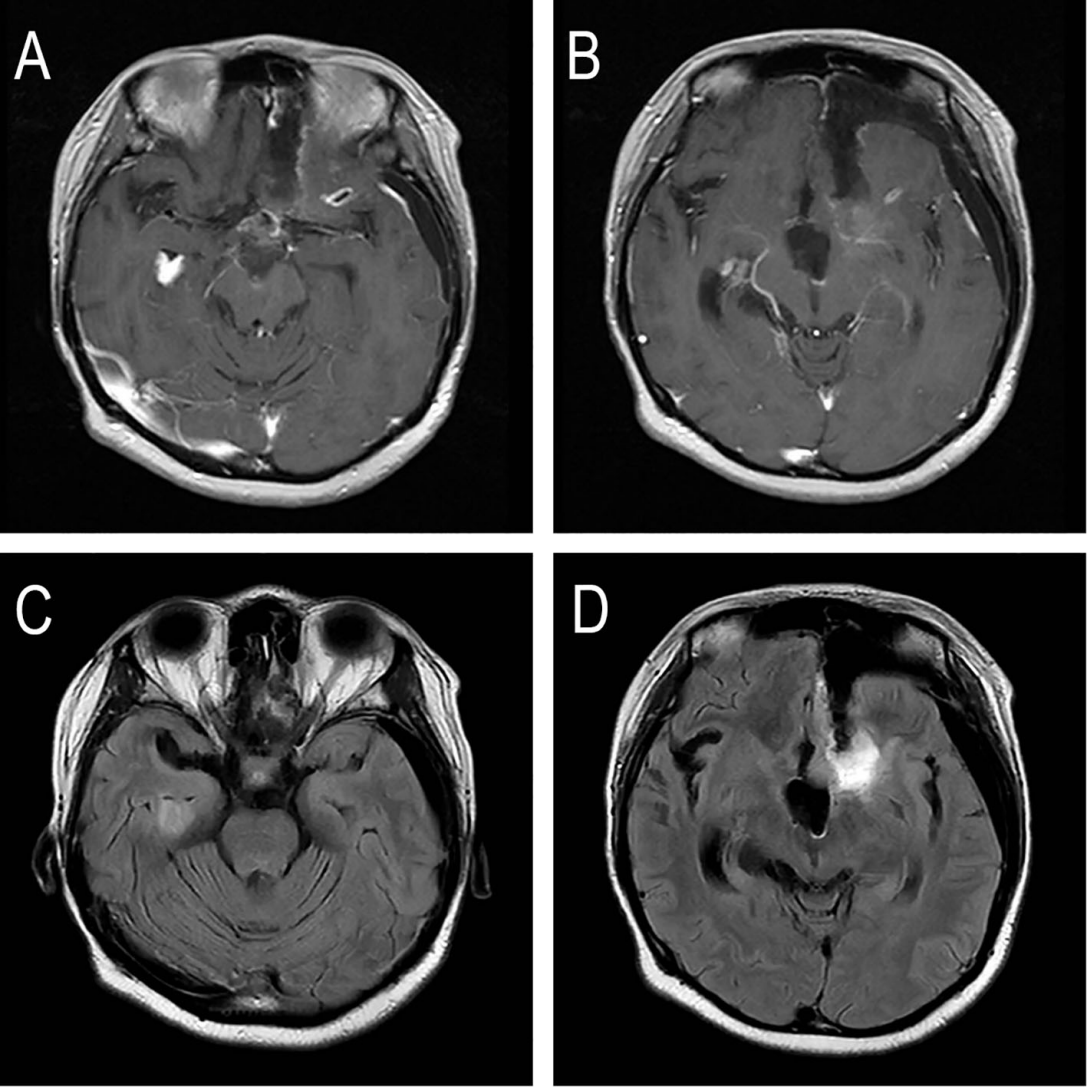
The patient’s MRI before re-radiotherapy. (A, B) T1-weighted contrast-enhanced MRI showing linear enhancement in the surgical cavity and the inferior horn of the right lateral ventricle. (C, D) T2-FLAIR MRI showing linear enhancement in the surgical cavity and the inferior horn of the right lateral ventricle.
